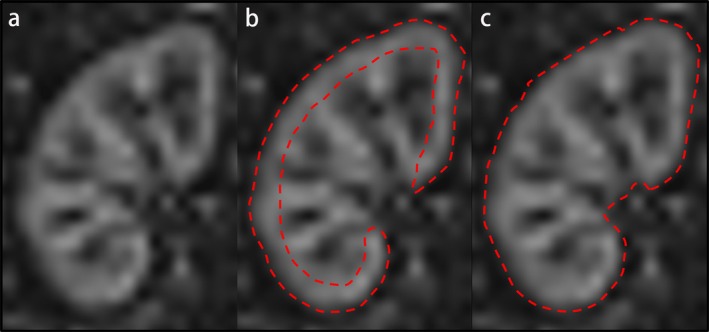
Panel (a) is the original image without regions of interest. The regions of interest used to measure RBF in the renal cortex (b) and the entire kidney (c) on ASL‐MRI images are outlined in red dashed lines. ASL‐MRI: Arterial spin labeling magnetic resonance imaging; RBF: Renal blood flow.
